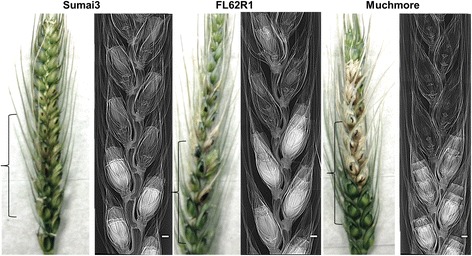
Images of diseased and healthy florets in the spikelets of wheat cultivars using phase contrast X-ray imaging at 4 days after inoculation with FHB. The spikelets were kept inside a 18 mm diameter falcon tube and X-ray images were recorded at 18 keV using a 8.75 μm resolution detector. Brackets indicate the imaged part of the wheat spike. Scale bars indicate 1 mm.
